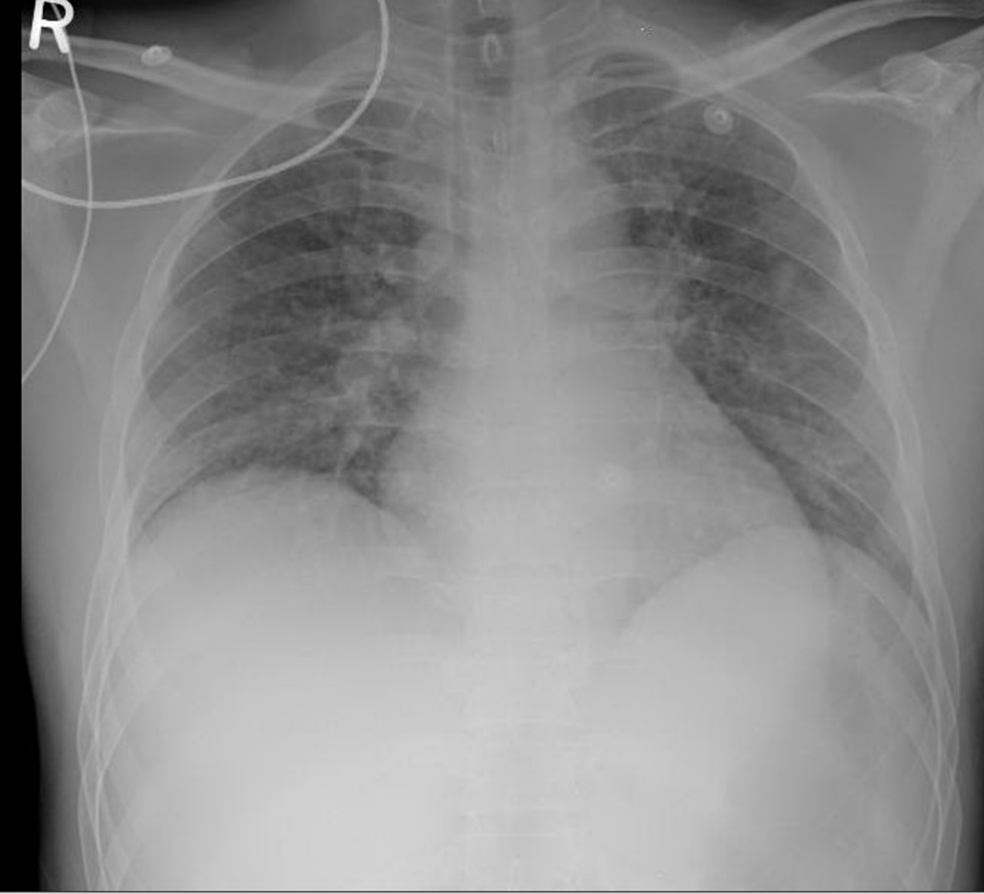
Chest X-ray showing bilateral fluffy infiltrates
